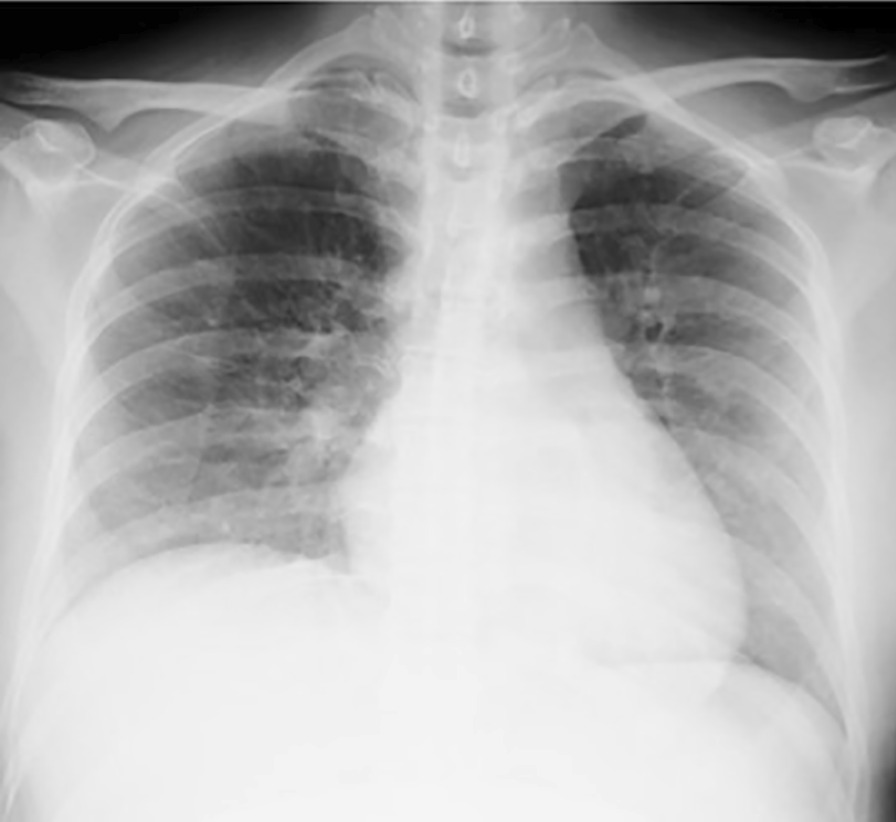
The chest X-ray taken in a sitting position after the patient developed dyspnea. Enlarged pulmonary vascular shadows indicated pulmonary edema
